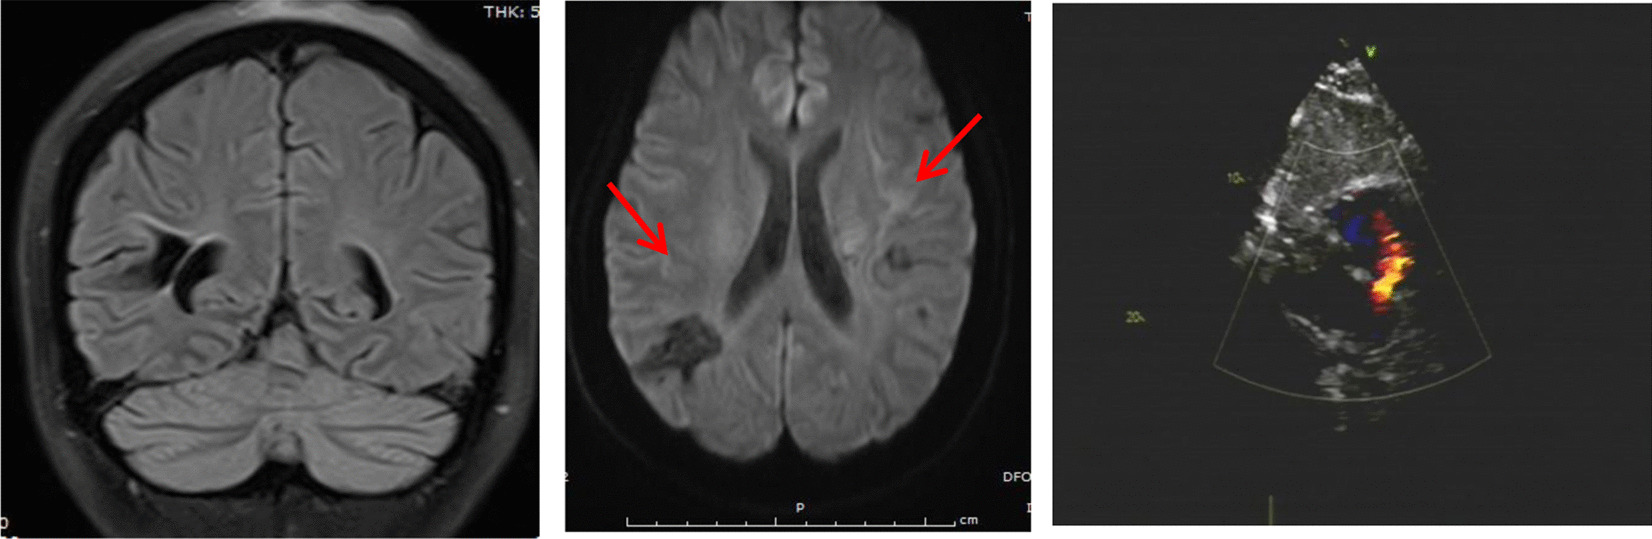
Example of a case with PFO and multiple brain ischemic lesions. One Tibetan female patient with age 25 years, resident in an area with 3850 m above sea level, is long-term suffering from headaches and dizziness. Left panel: brain MRI shows low-intensity signal by a coronal section on FLAIR. Middle panel: DWI shows multiple low-intensity lesions in both hemispheres, which are consistent with old infarcts. Right panel: echocardiography imaging of the heart. Arrow points to PFO with diameter of 5.5 mm
